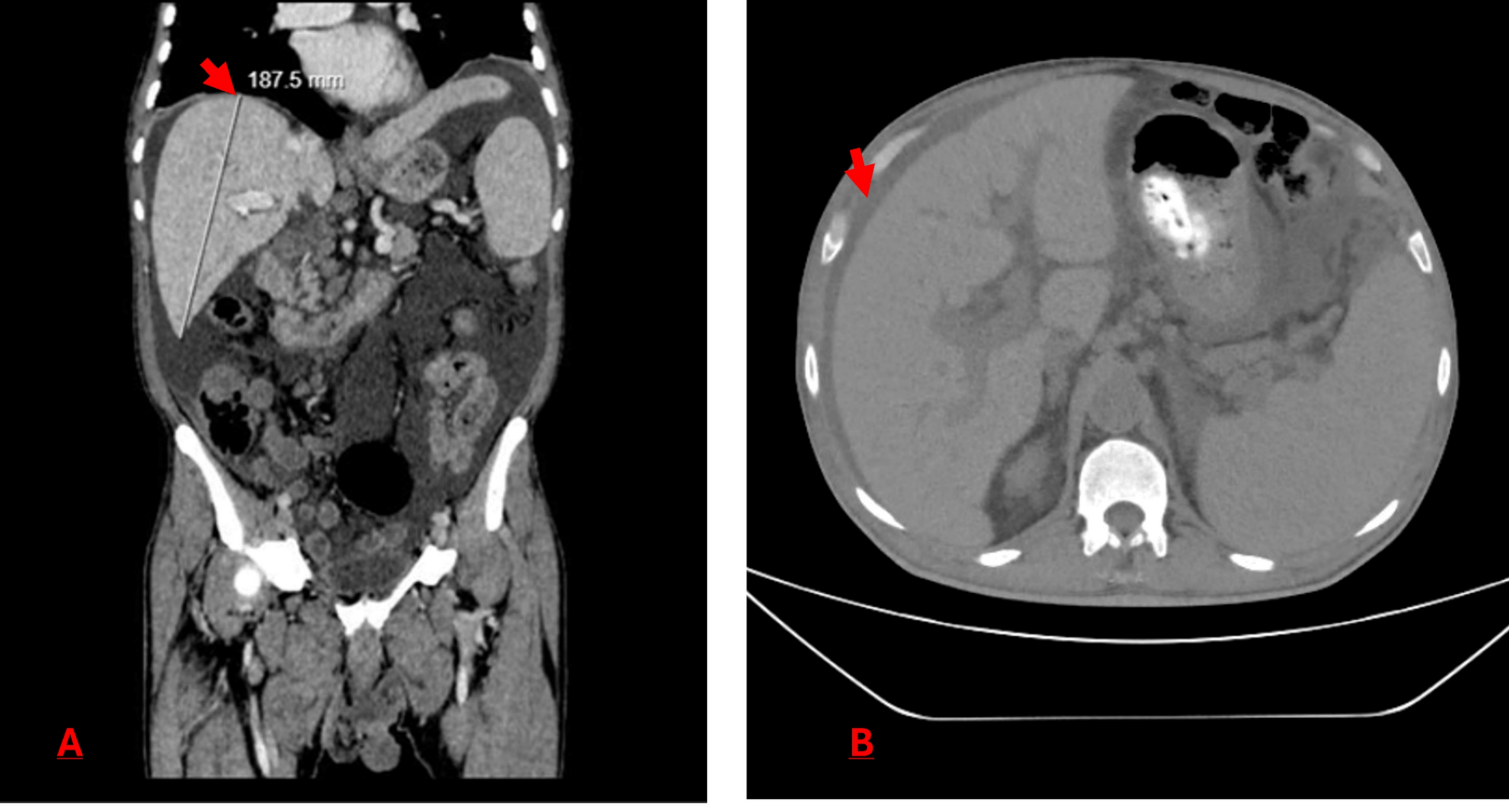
Contrast-enhanced computed tomography of the abdomen. (A) Coronal reconstruction showing marked hepatomegaly (craniocaudal length: 18.7 cm; arrow). (B) Axial image demonstrating perihepatic ascites (arrow).
